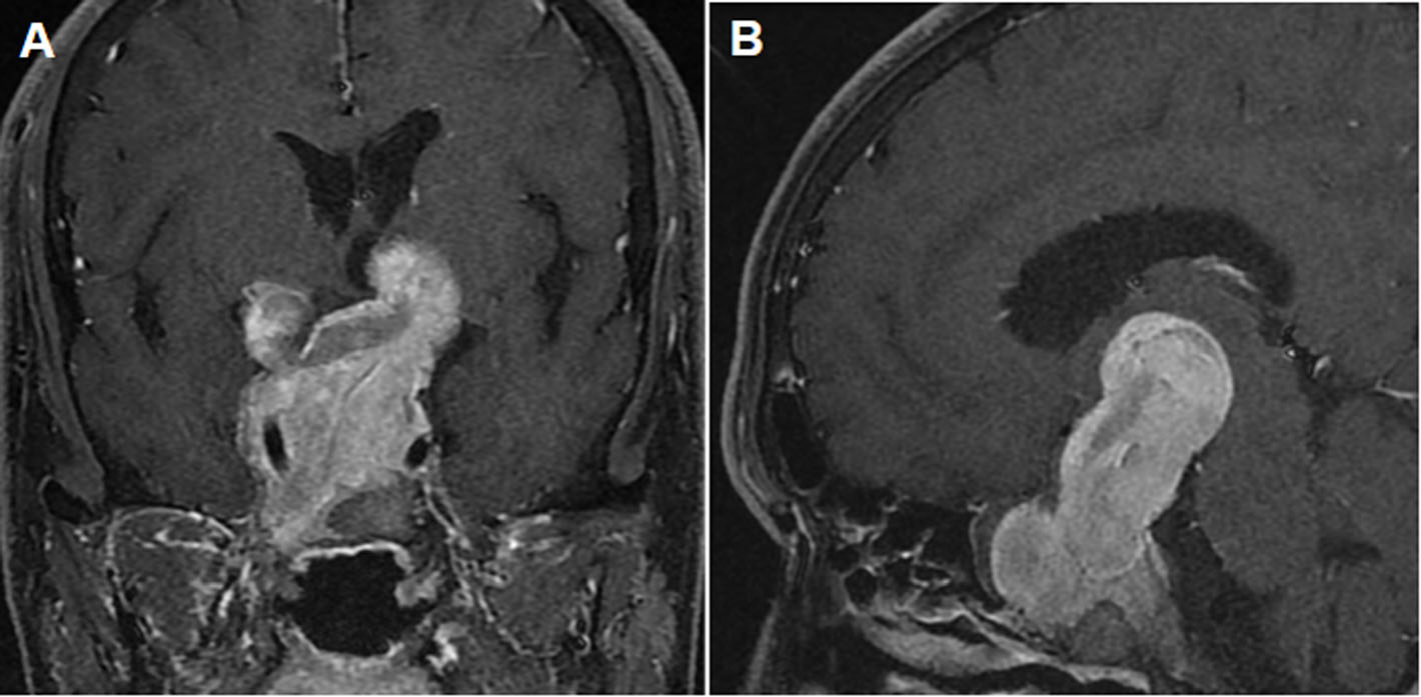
Sella MRI revealed a 7.0 × 6.2 × 5.1 cm sized lobulated heterogeneously enhancing pituitary tumor with supra-sellar expansion. A Coronal post-contrast T1 weight image; B Sagittal post-contrast T1 weight image
